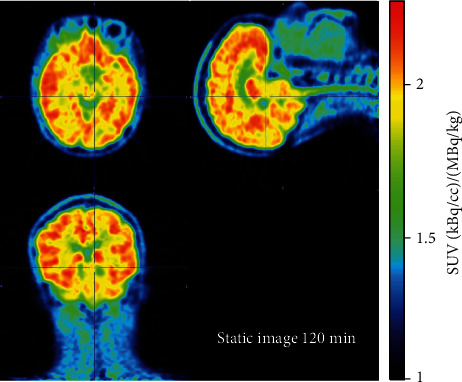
120-minute static image (SUV units) for the first participant (P1), injected with [^18^F]SDM-4MP3, scaled to maximum observed SUV.
